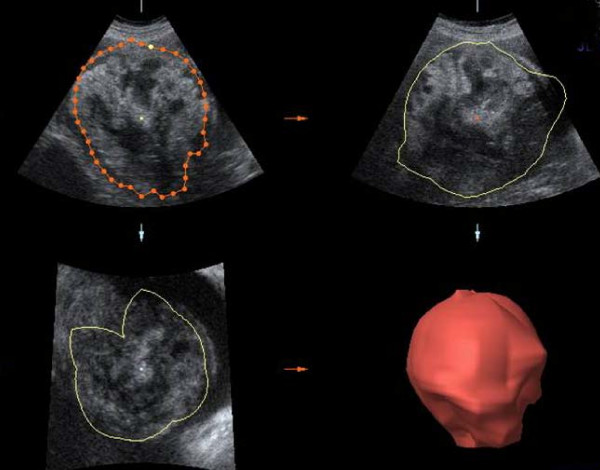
Ultrasonographic findings of placenta accreta left *in situ *with measurements to estimate the total volume during follow-up.
